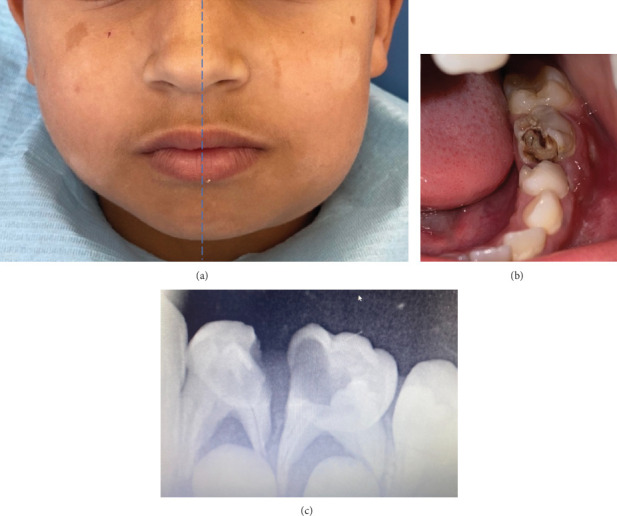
(a) On examination, the face appeared asymmetrical with a swelling in the left cheek. Overlying skin was of normal color. Mouth opening was 25 mm. (b) Endobuccal view showing Teeth 74 and 75 with twin cavities and a buccal suppurated collection. (c) Retroalveolar radiography of Teeth 74 and 75 showing the pulpoperiodontal implications.
